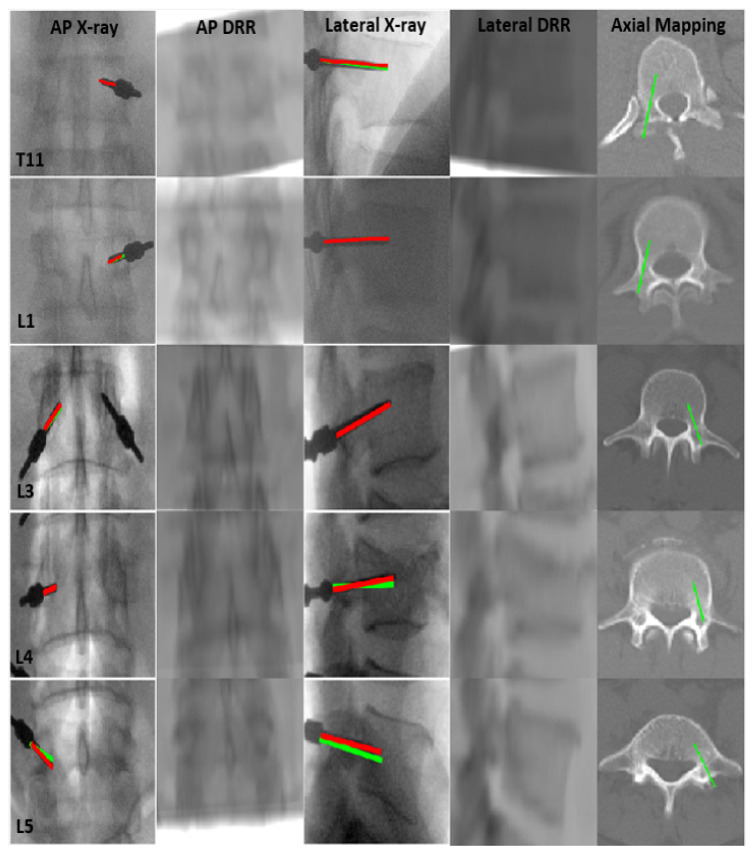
Clinical validation of pedicle screw trajectory registration: Red and Green lines in Columns 1 and 3 are the center axis of true and reprojected trajectories displayed on AP and lateral radiographs, respectively. Columns 2 and 4 display DRR images generated at the optimal anatomical landmark registration point in the AP and lateral planes, respectively. Column 5 displays axial trajectory mapping on pCT at optimum registration point of different vertebral levels—Source: Orthopaedics and Radiology Department—KMC Manipal.
